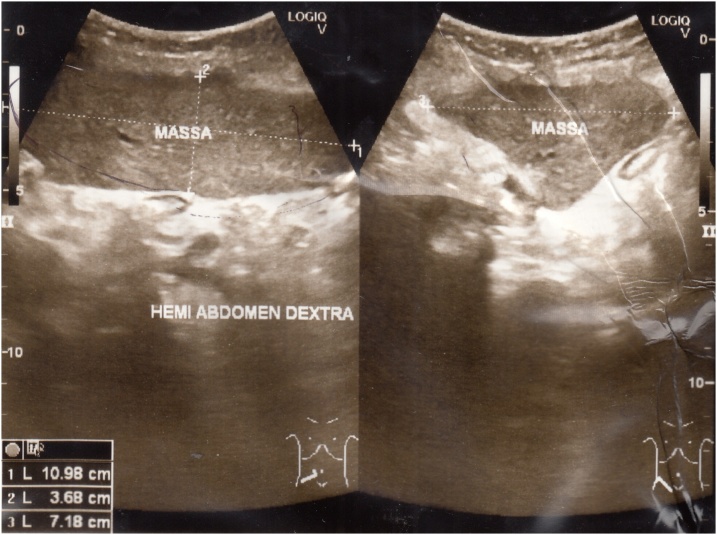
Ultrasonography of the abdomen showing a heterogeneous mass within the right abdominal cavity.
